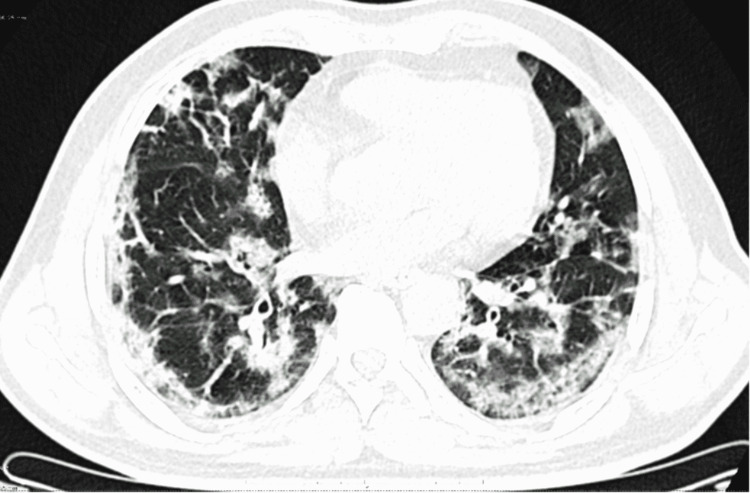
Axial CT section of the chest showing parenchymal bands and peripheral subpleural predominant distribution of opacities in a patient with COVID-19 pneumonia
